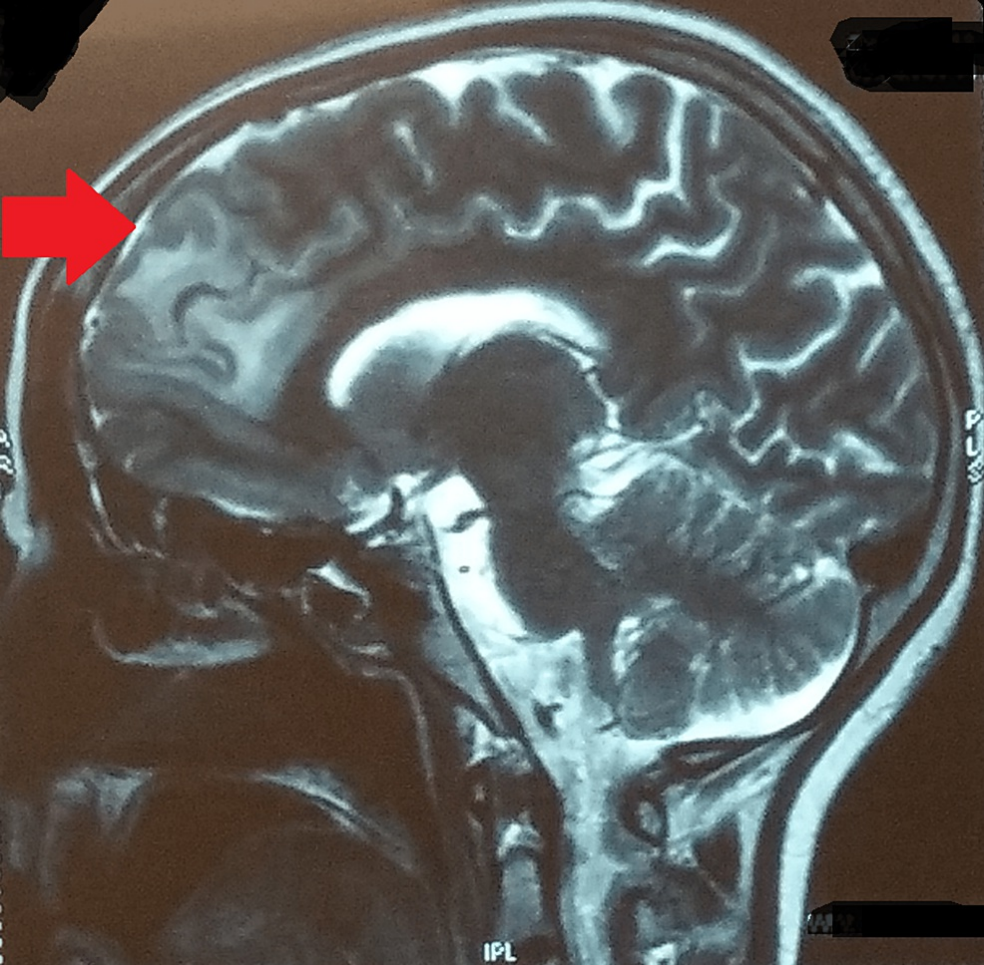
MRI brain showing left parasagittal frontal lobe hyperintensity MRI: magnetic resonance imaging
